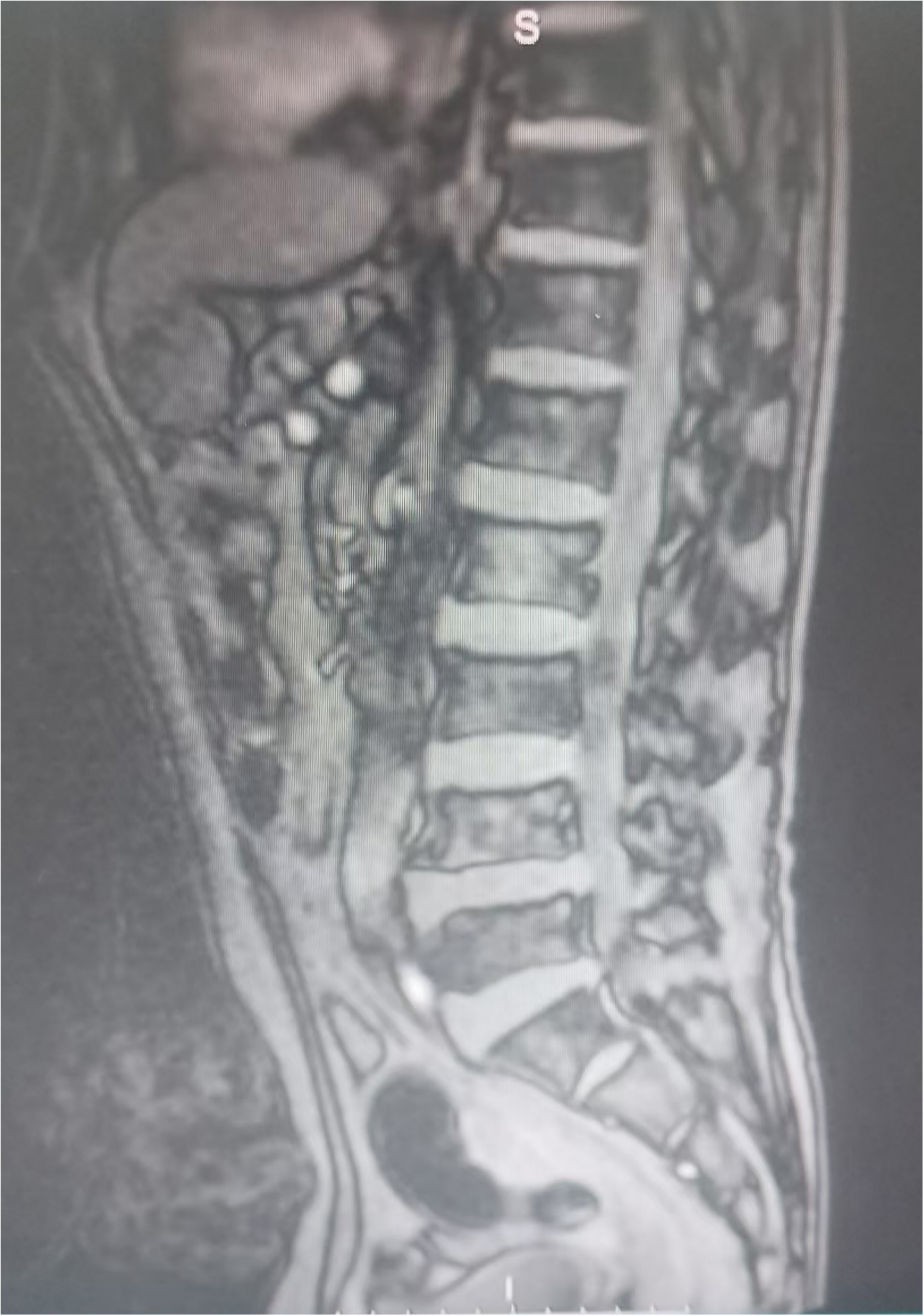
The patient’s spinal MRI showed no abnormalities.
